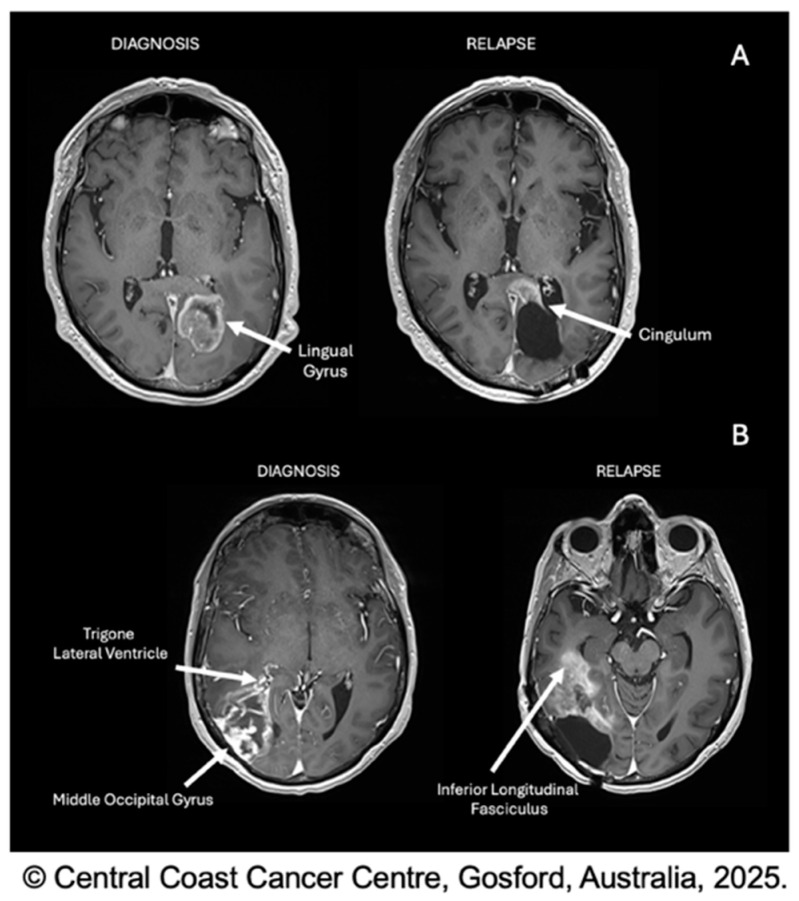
Axial T1-weighted gadolinium-enhanced MRI; examples of initial infiltration and progression patterns. (A) Medial tumour with progression involving the cingulum; (B) lateral tumour infiltrating towards the trigone, with progression involving the inferior longitudinal fasciculus.
